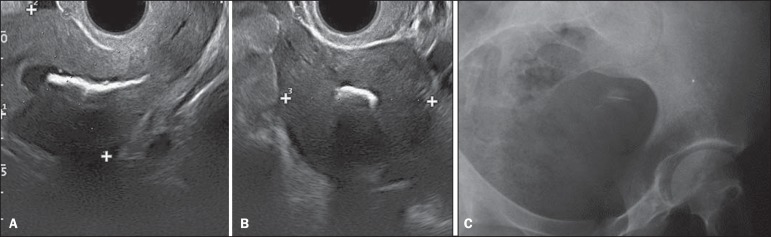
A,B: Transvaginal ultrasonography demonstrating hyperechoic
